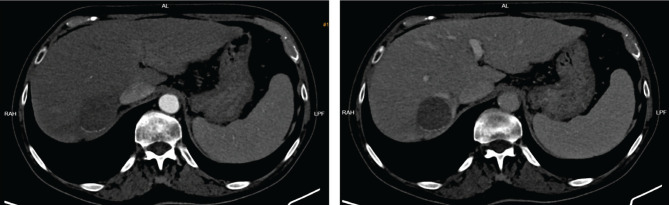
CT scan with intravenous contrast at 12 months post-procedure (arterial and venous phase) – complete tumor necrosis.
